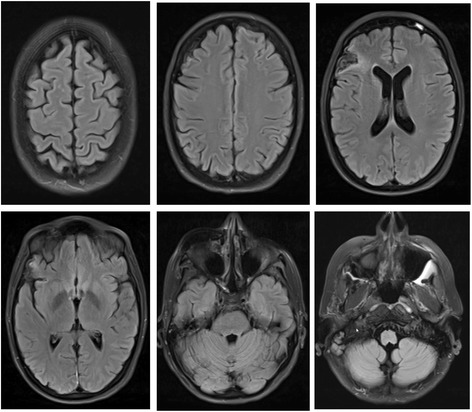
T2-weighted MR of the Head 17 Days after Cardiopulmonary Resuscitation. Selected t2-weighted axial slices of the head 17 days after cardiopulmonary resuscitation. Except for a, most likely preexisting, small cortical defect of the right frontal lobe (upper row, right image) the MRI of the brain shows normal findings
